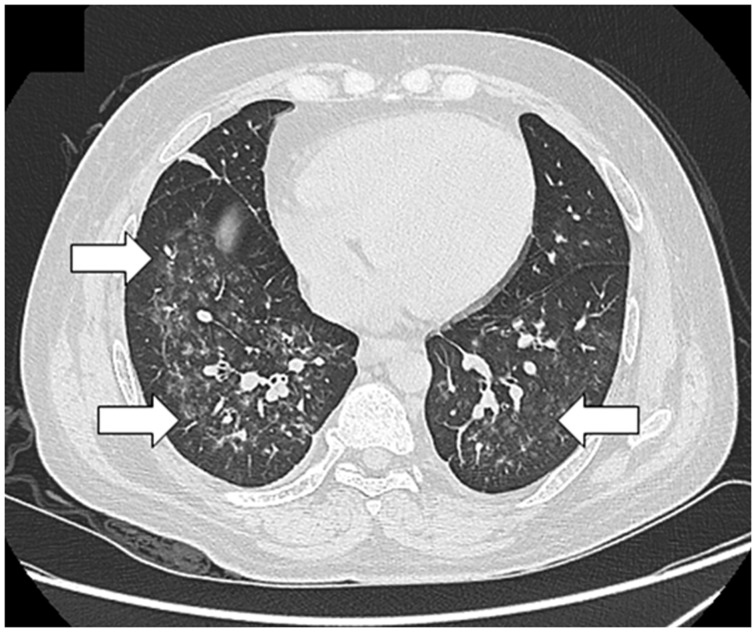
Pneumocystis jirovecii* pneumonia. High-resolution CT scan of a lung window in a case of *Pneumocystis jirovecii* pneumonia showing bilateral perihilar ground glass opacities and few centrilobular nodules.
